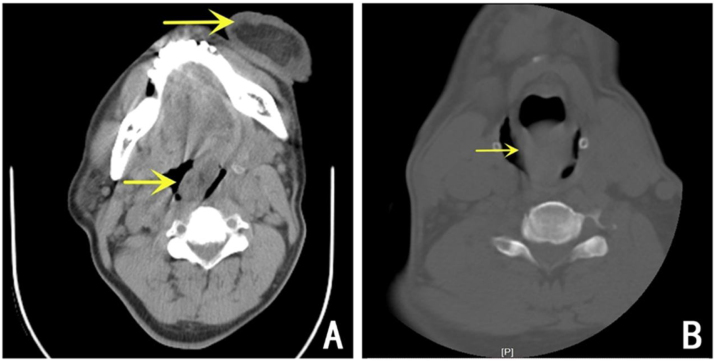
(A) Preoperative CT showed that the upper respiratory tract in the oropharynx and throat was occupied by soft tissue density and extended into the mouth. (B) Preoperative CT showed a huge, large mass in the laryngopharynx with visible dilatation of the esophageal entrance.
